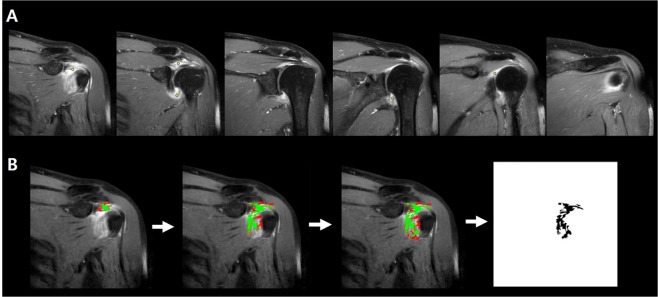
Semiautomatic segmentation of the regions of interests (ROIs) using the Fast Marching method of Level Set plugin (https://imagej.net/Level_Sets) in Fiji, an open-source image processing package based on Image J. (A) Before image segmentation, all oblique coronal fat-suppressed enhanced T1-weighted images were imported to the Fiji software and the image dynamic was reduced from 16-bit to an 8-bit type to match the requirements of the used software. In the Fiji environment, the operator manually selected several seed points on the capsulo-synovial enhanced portion in the serial MR image stack. Capsulo-synovial enhanced portion was defined as the area where signal intensity was enhanced and regarded as pathologic condition. (B) Then, we went to the Level Sets plugin dialog and selected the Fast Marching option. Clinking on OK button, Fiji automatically updated progress window (the first, second and third figures) and, after completion, showed a result window (the fourth figure). The segmented area was shown in green in the progress window and black in the result window. To detect segmentation boundaries, the Fast Marching method algorithm expands from a seed point to the segmentation boundary until it encounters a pre-set grey value difference in the pixel intensity. While growing the region of interest (ROI), it constantly calculates the difference of the current selection to the newly added pixels and either stops if it exceeds a pre-selected grey value difference. The grey level value of each pixel is in the range of 0-255. In this study, the threshold of grey value difference between boundary pixel and the seed point was pre-set with 50, which determines the stopping point for the expansion.
